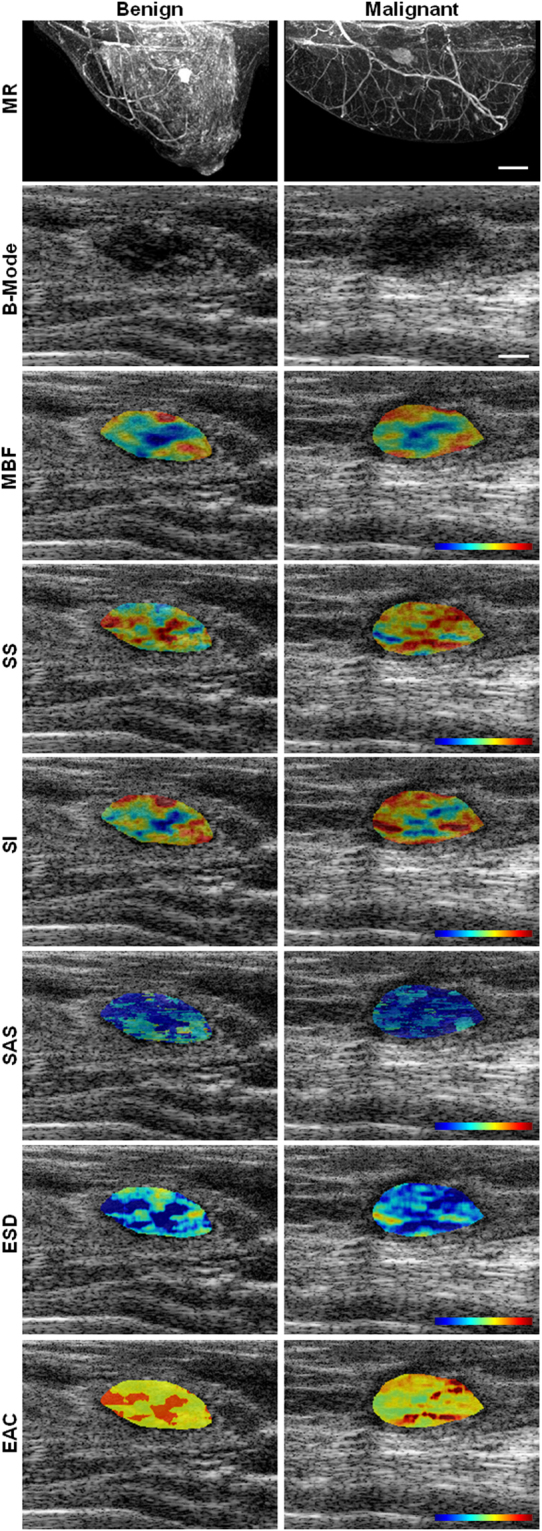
Representative MR and ultrasound B-mode images with QUS parametric overlays of MBF, SS, SI, SAS, ESD, EAC obtained from a benign and a malignant lesion. The color bar represents a scale encompassing 20 dBr for MBF, 5 dBr/MHz for SS, 30 dBr for SI, 3 mm for SAS, 160 µm for ESD, and 50 dB/cm^3^ for EAC. The scale bar represents 2 cm and 5 mm in MR and ultrasound images, respectively.
